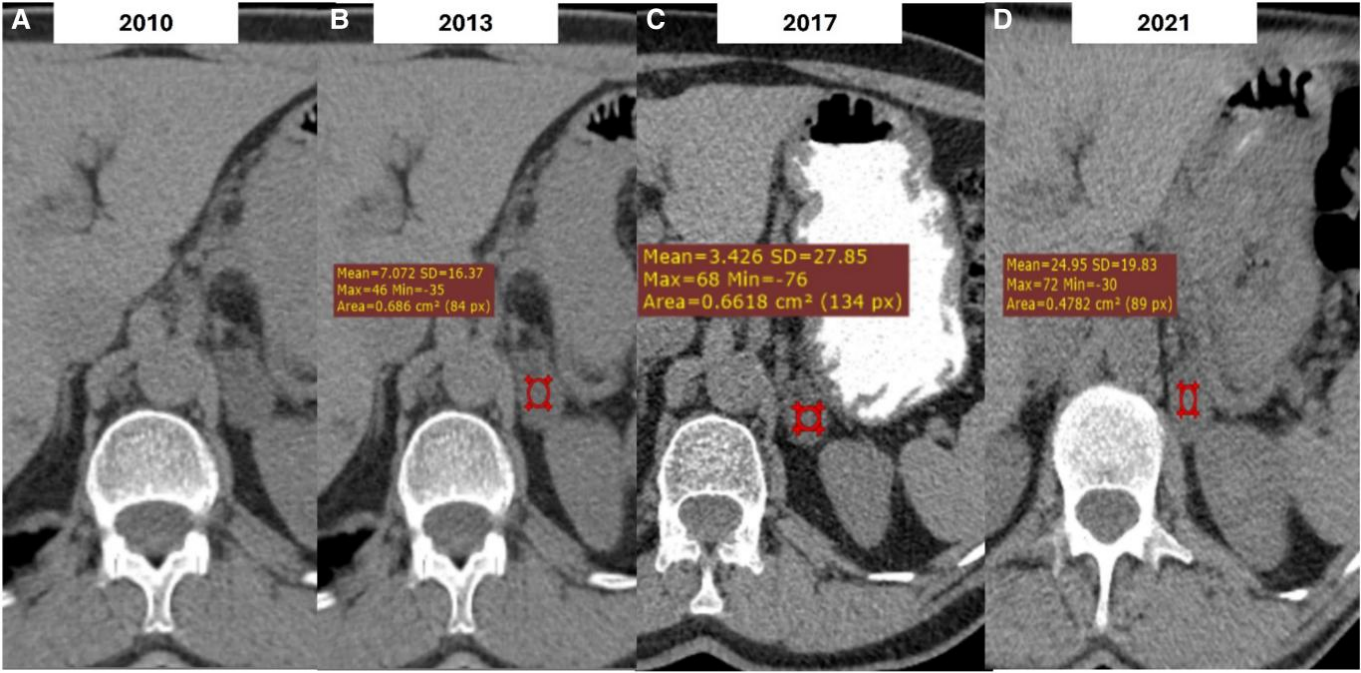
Axial unenhanced Computerised Tomography (CT) image of the upper abdomen demonstrates a nodular left adrenal lesion of 3 cm in size with low attenuation values <10 HU consistent with adenoma (A). Follow-up CT images of the same patient show no significant change of the lesion's size or density with mean ± SD density values equal to 7 ± 16.37 HU (max = 46, min = −35) (B) and 3.4 ± 27.85 HU (max = 68, min = −76) (C). On the follow-up CT performed one year before adrenocortical carcinoma (ACC) diagnosis the lesion remains stable in size but demonstrates higher mean ± SD density values equal to 24 ± 19.83 HU (D). Red circle: region of interest (ROI), HU: Hounsfield unit, Mean: mean HU, Max: maximum HU, Min: minimum HU, SD: standard deviation, Area: area of a square (cm^2^).
